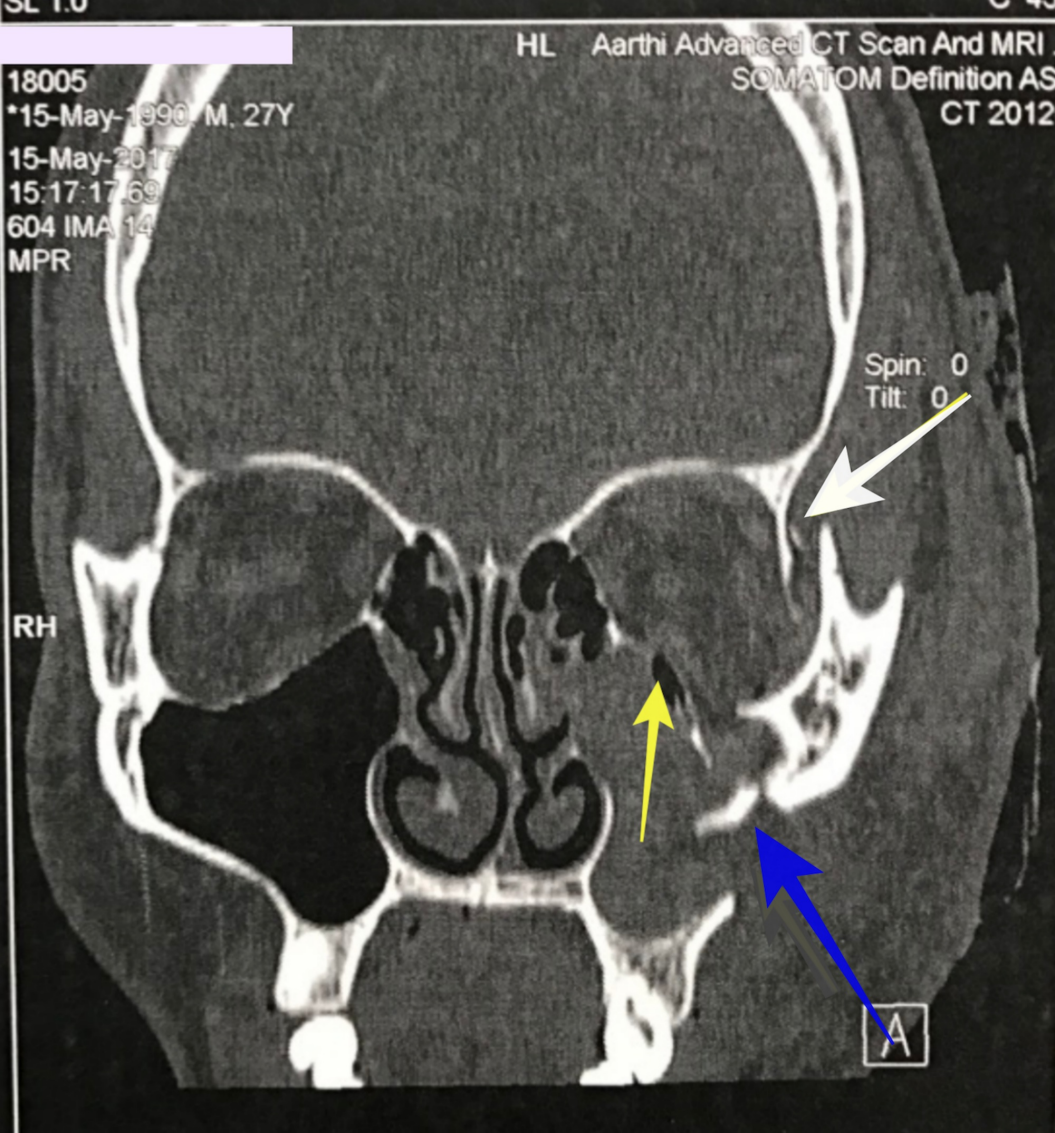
Computed tomography scan (coronal view) demonstrating fracture at left front zygomatic region (white arrow), orbital floor involving the infraorbital rim (yellow arrow) along with fracture of the left zygomatic maxillary buttress region (blue arrow).
